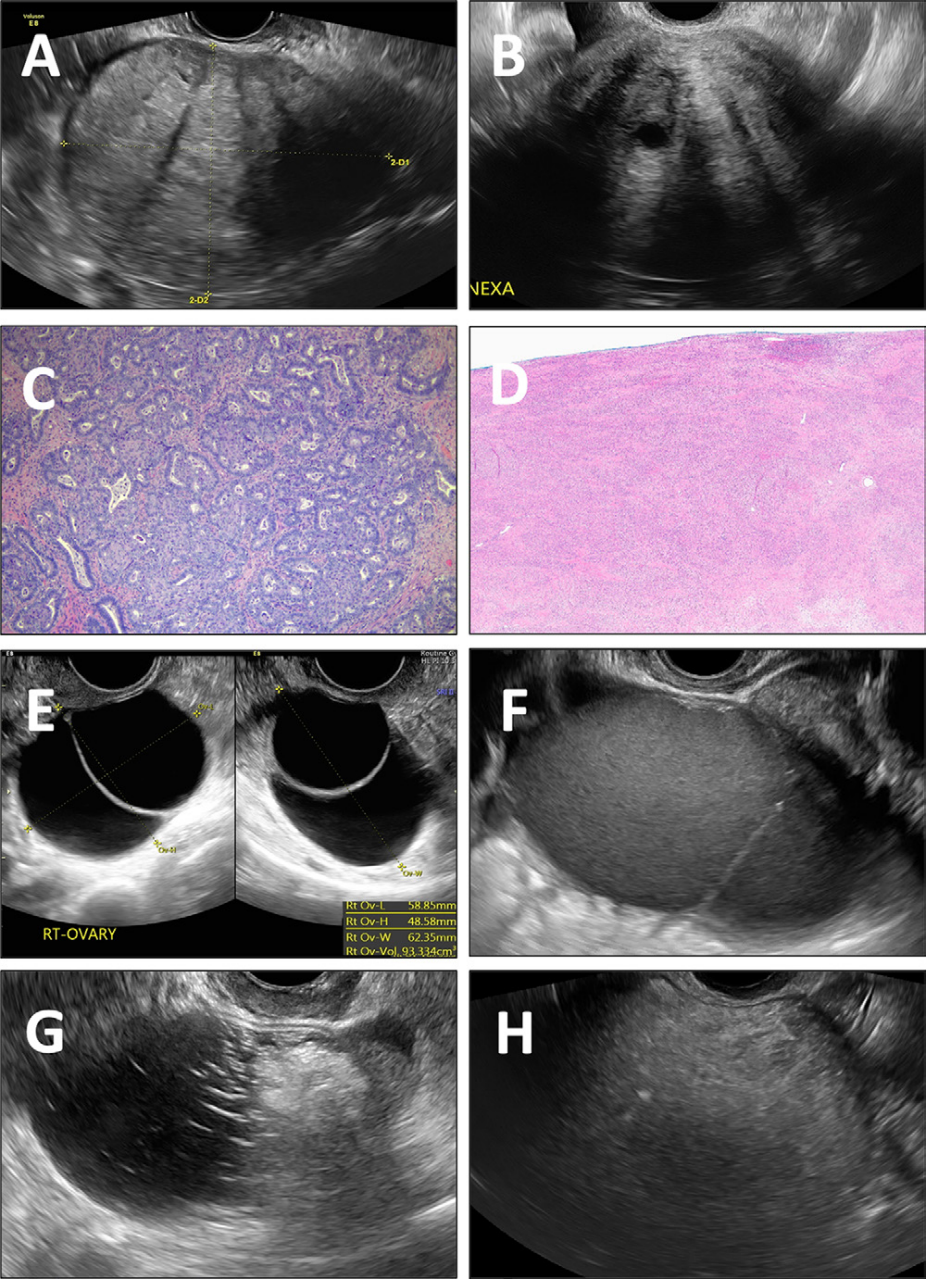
Sonographic features characterized in Ovarian-Adnexal Reporting and Data
